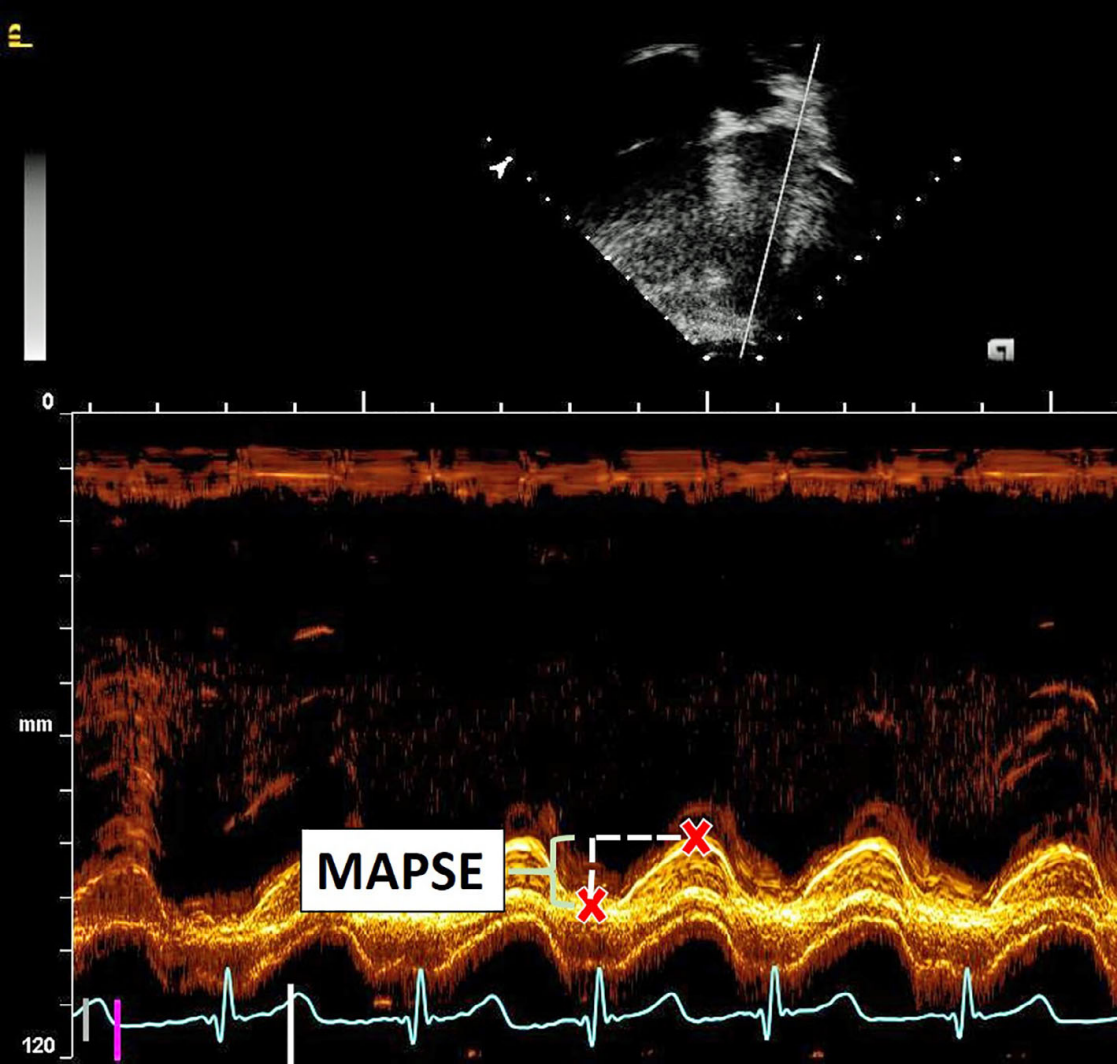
Four chamber echocardiographic image M‐mode across the lateral edge of the mitral valve annulus. The red letter “x” marks the position of the lateral wall at end diastole and at its highest point at the time of mitral valve closure. The height between these two positions is MAPSE. MAPSE, mitral annular plane systolic excursion; mm, millimeter
